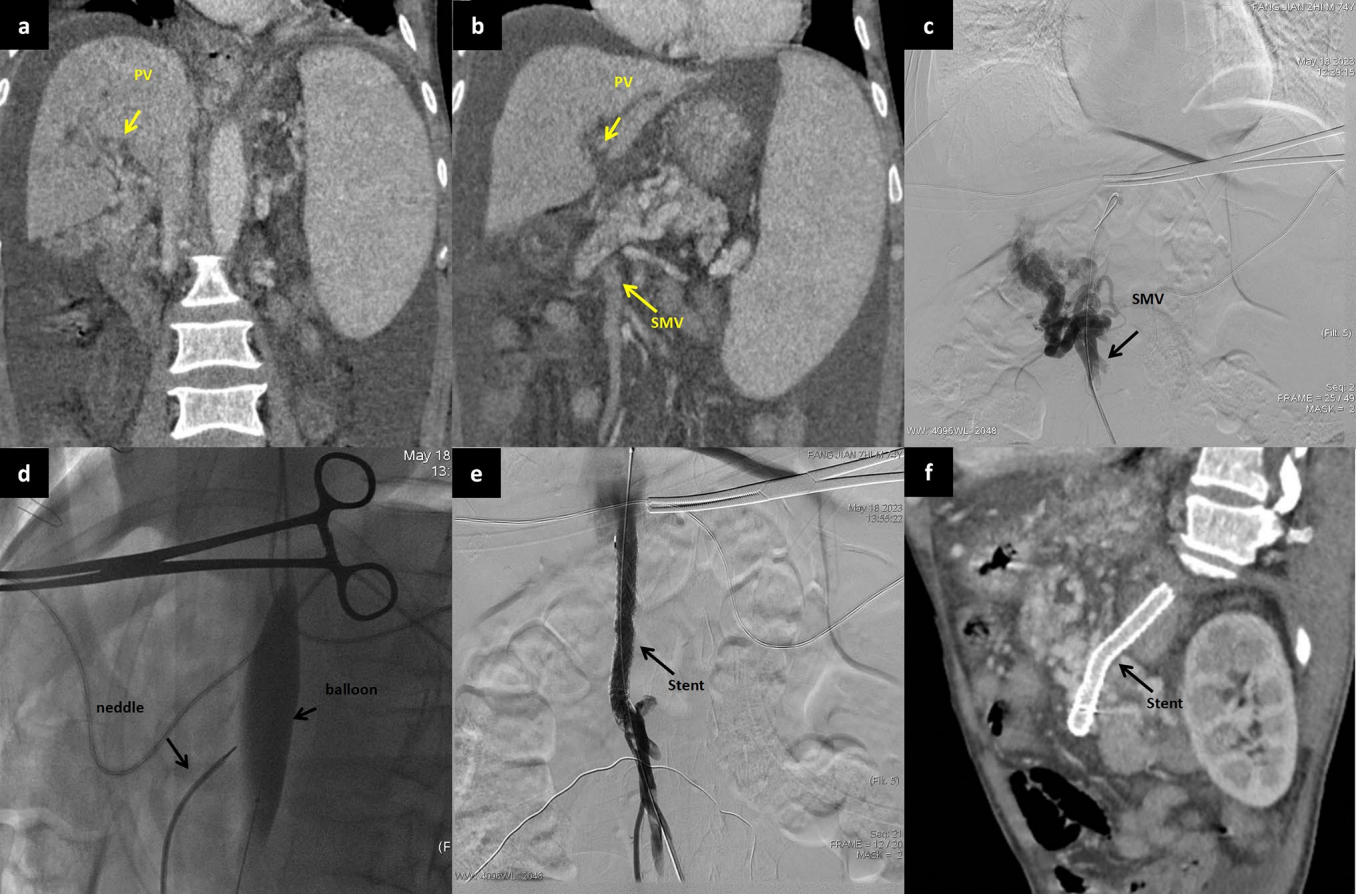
A 74-year-old male patient, who has been dealing with liver cirrhosis for 30 years, has been suffering from recurrent episodes of esophagogastric variceal hemorrhage and recurrent ascites for the recent three years. Previous interventions, including ESVD and LVP, have proven to be ineffective. a–b The multiplanar reconstruction from the spiral-enhanced CT scans demonstrated cavernous transformation of the portal vein, with patent mesenteric veins. c The transabdominal mesenteric venography reveals a patent mesenteric venous system and the presence of extrahepatic collateral vessels. d Utilizing a 14 G metal tube, part of the RUPS-100 apparatus, the tube was directed to the proximal region of the SMV with the aid of a guidewire. Following this, a pre-inserted balloon located in the posterior segment of IVC was successfully punctured. e A stent was successfully placed between SMV and IVC. f The spiral-enhanced CT with multiplanar reconstruction images show that the stent is patent at 1 month after TEPS. *PV* portal vein, *IVC* inferior vena cava, *SMV* Superior mesenteric vein, *ESVD* Endoscopic selective varices devascularization, *LVP* large-volume paracentesis
